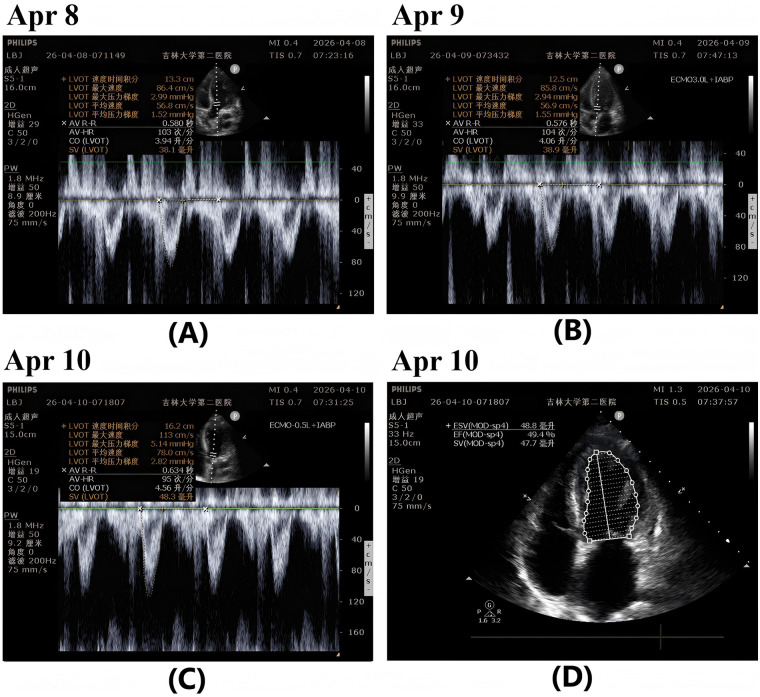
Serial bedside echocardiographic assessment of cardiac function after ECMO weaning. (A–C): From April 8 to April 10, the velocity-time integral was measured using pulsed-wave Doppler of the left ventricular outflow tract, and SV and CO were calculated. (D) On April 10, left ventricular volumes were measured using the single-plane modified Simpson method in the apical four-chamber view, and LVEF was calculated.
